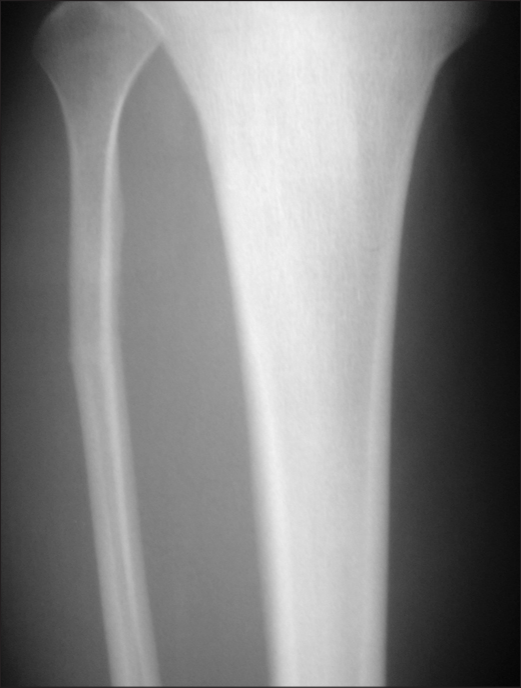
A plain tibial anteroposterior radiograph of a 21 year-old man shows a proximal fibular stress fracture with some lateral angulation.
